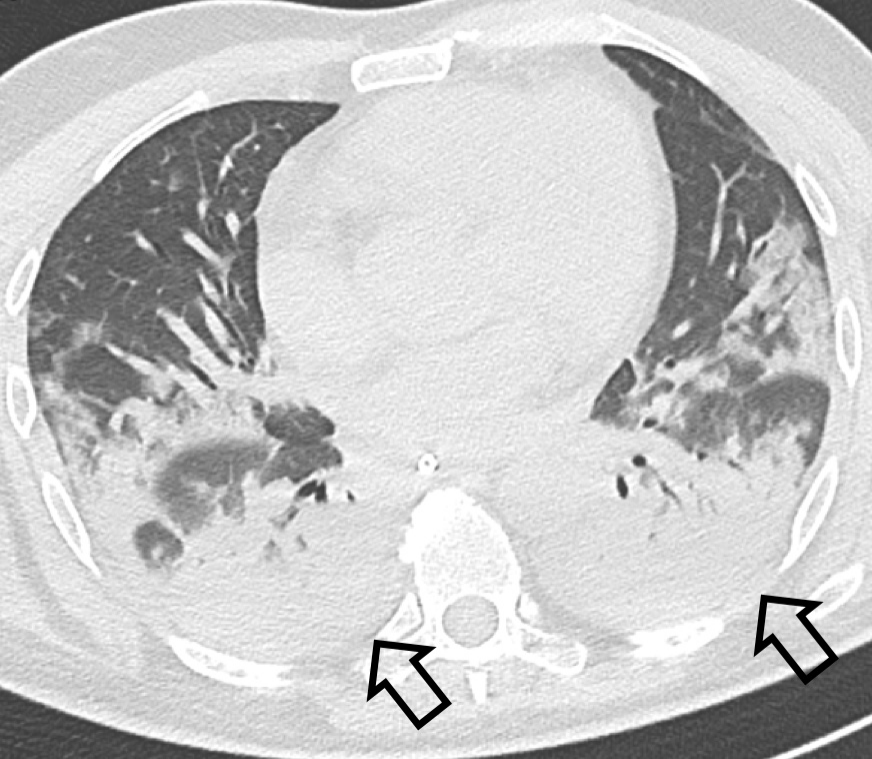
Axial CT image without contrast showing dependent subpleural atelectasis (open arrows).
